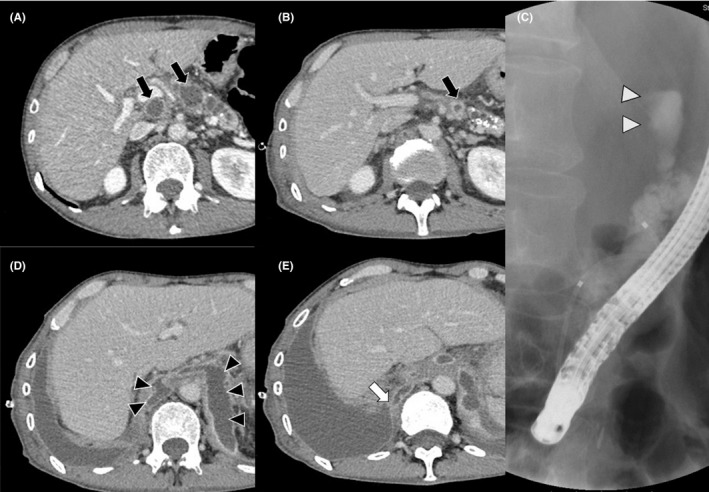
A, Nine months prior to patient admission, a pancreatic pseudocyst (black arrow) was observed on a computed tomographic scan. B, On admission, the pseudocyst had shrunk (black arrow). C, Endoscopic retrograde cholangiopancreatography showed the leakage of contrast medium from the pancreatic duct (white arrowhead). D, The fluid under the diaphragm from the pancreatic pseudocyst was observed on a computed tomographic scan. The fluid traversed the front of the aorta into the posterior mediastinum (black arrowhead). E, A computed tomographic scan showing the fluid approaching the right thoracic cavity through the pleura (white arrow)
